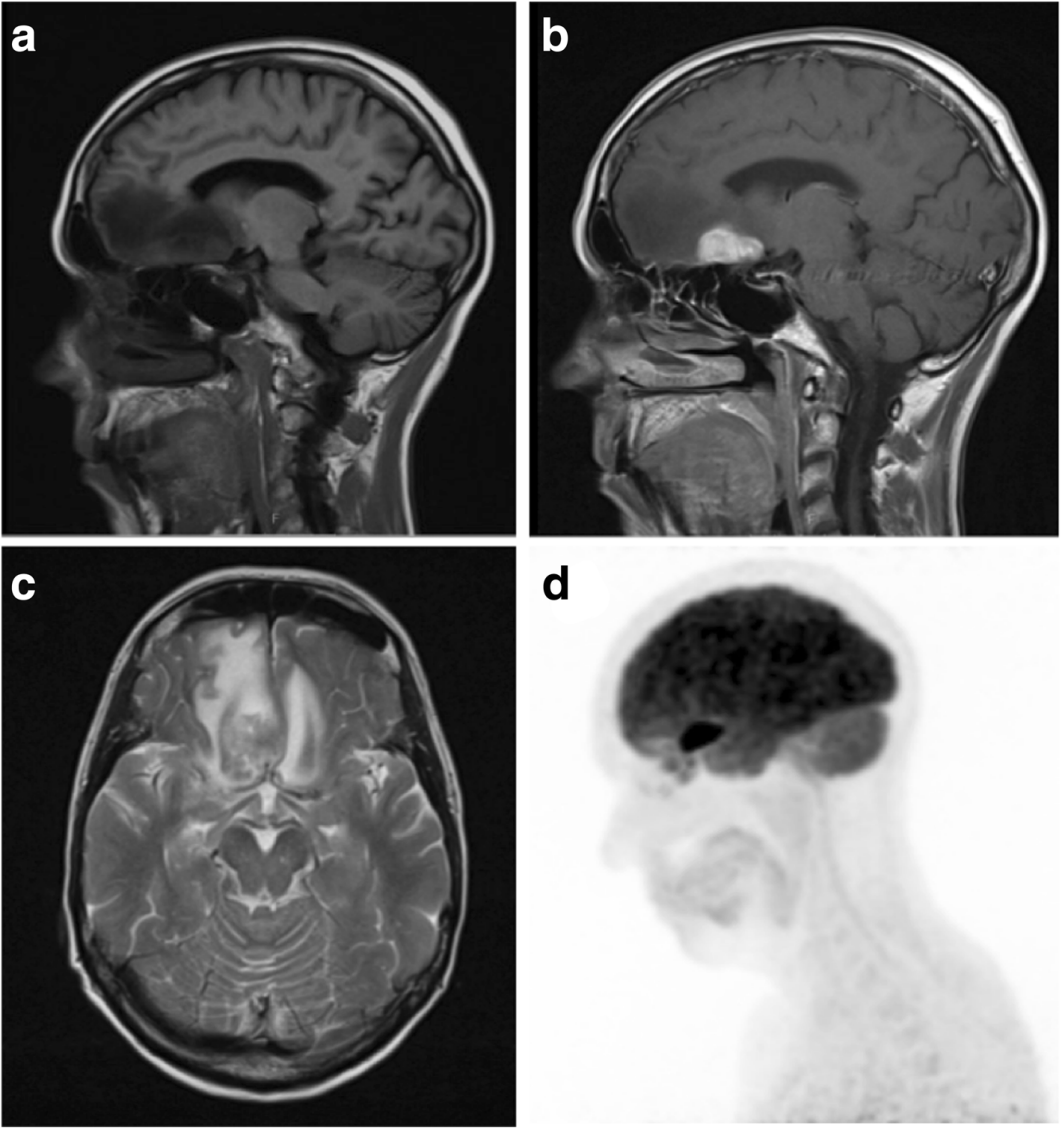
Lymphomatous deposit. a, b Pre- and post-contrast sagittal T1-weighted MR images showing a solitary extra-axial lymphomatous deposit with a wide dural base and avid enhancement. It is lobulated, and its border is ‘fluffy’ and less distinct than that of the typical meningioma. There was no skull hyperostosis or intratumoural calcification on CT imaging. c Axial T2-weighted MR image of the same lesion showing marked vasogenic oedema. d Maximum intensity projected FDG PET image demonstrates avid tracer uptake within the lesion
